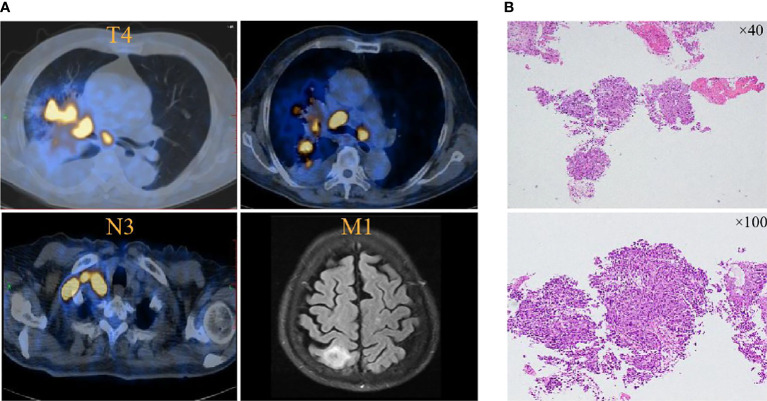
PET and brain MRI images at initial diagnosis. (A) Lung cancer is present in the hilar angle of the right lung before pembrolizumab treatment in November 2021. (B) Tissue biopsy showed squamous lung cancer.
